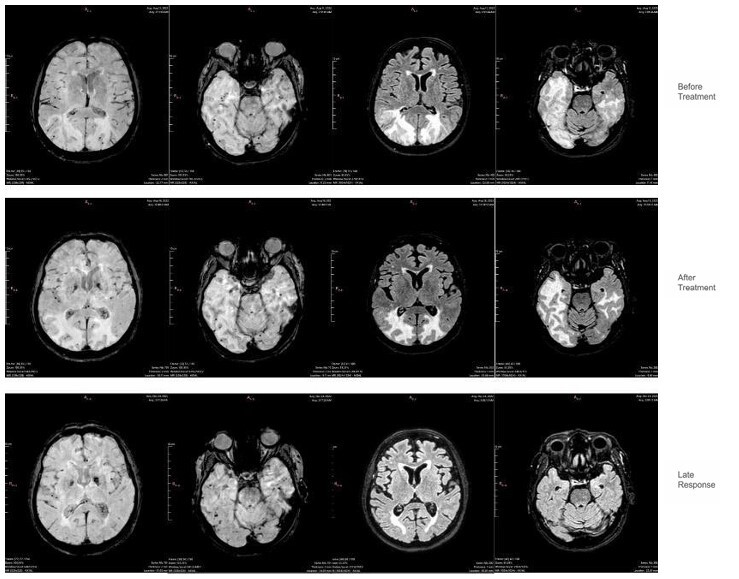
Images related to Case 2 show, from left to right, two susceptibility-weighted imaging- MRI slices and their corresponding FLAIR-weighted slices, highlighting that the hyperintense signal in the latter corresponds to areas with the highest concentration of hypointense signals on the susceptibility-weighted imaging sequence, which in this case are suggestive of hemorrhage. The middle and lower images demonstrate post-treatment evolution and a delayed response.
